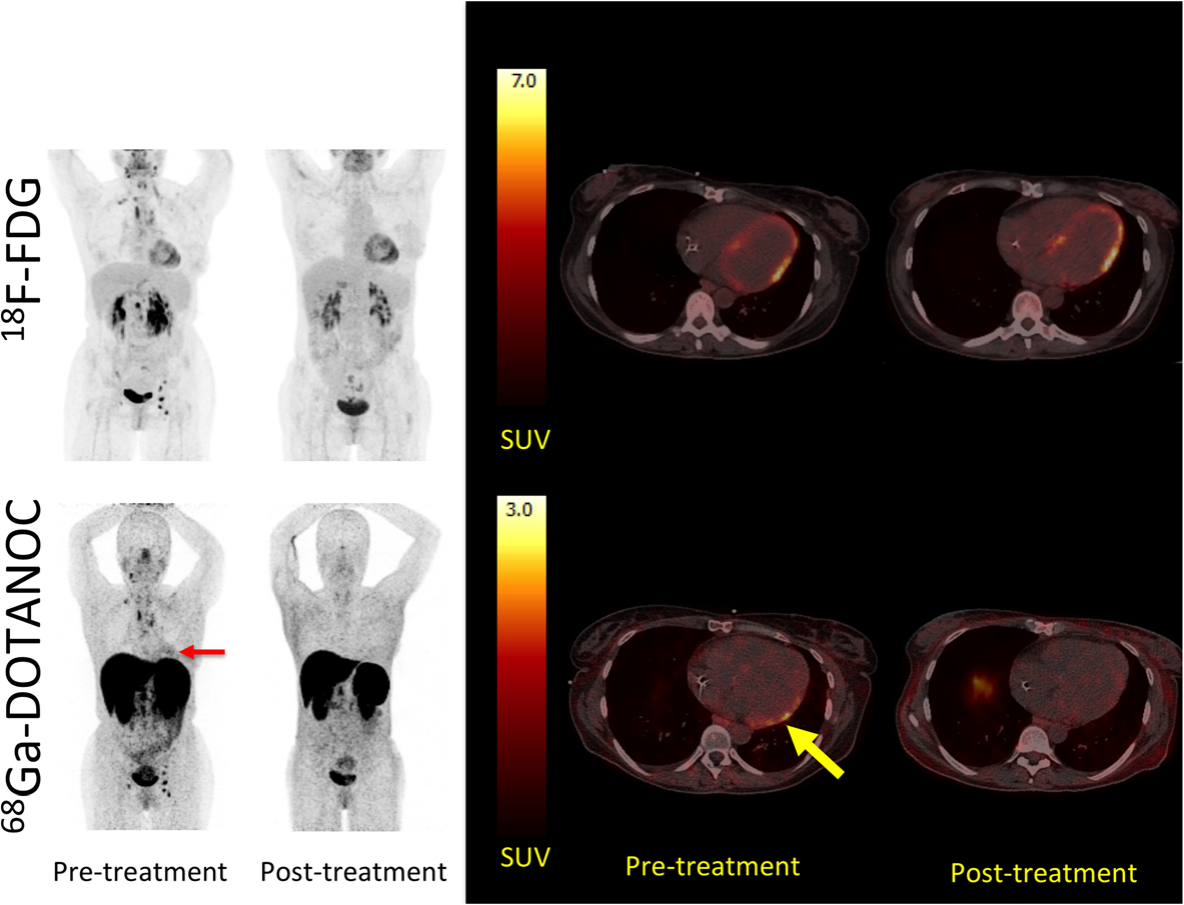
The effects of corticosteroid treatment on ^18^F-FDG and ^68^Ga-DOTANOC uptake in the cardiac region. *Left panel*: patient no. 4 (with CS according to the reference standard) was dual-tracer scanned before treatment with corticosteroids and after 6 months of high-dose prednisolone treatment (initially 50 mg tapering off to 37.5 mg). At diagnosis, there were multiple ^18^F-FDG and ^68^Ga-DOTANOC avid lymph nodes on both sides of the diaphragm as well as accumulation of ^68^Ga-DOTANOC in the cardiac region (*red arrow*) (SUVmax 2.64, target-to-background 2.54). The patient was rated as positive for CS on the ^68^Ga-DOTANOC PET/CT. *Right panel*: transaxial slices of the same patient where ^18^F-FDG uptake in the myocardium (*upper row*) is similar during both scans (focal on diffuse) whereas the ^68^Ga-DOTANOC accumulation (*lower row*) in the basal inferolateral wall (*yellow arrow*) is completely abolished after treatment
